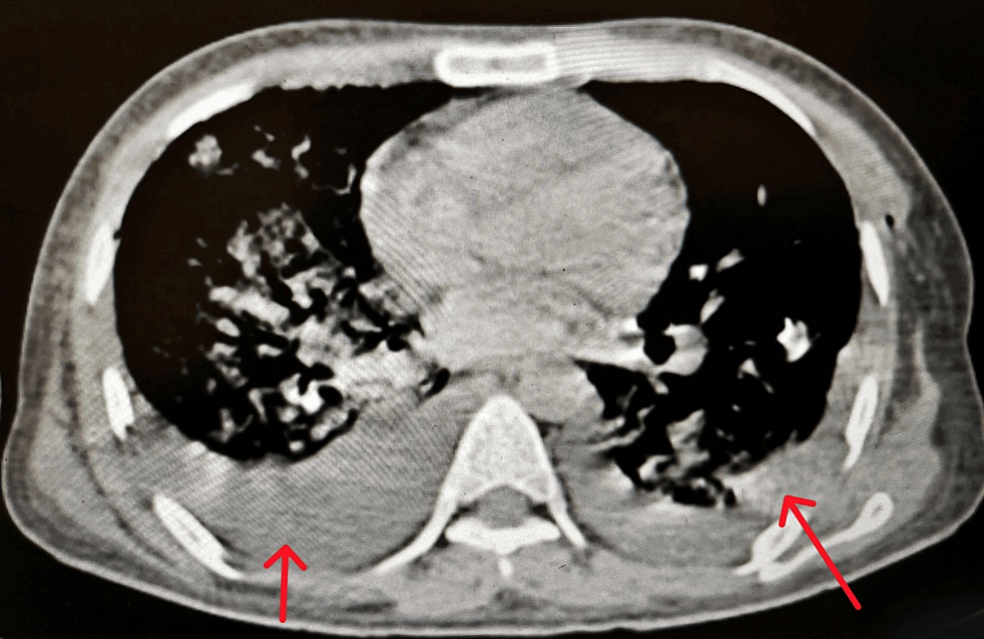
Mediastinal window of computed tomography showing bilateral moderate pleural effusion with collapse consolidation of underlying pulmonary parenchyma and bilateral basal atelectasis
